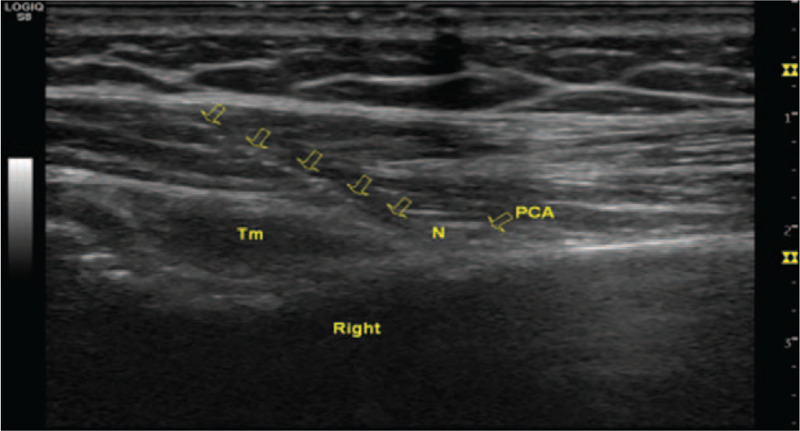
Axillary nerve block in quadrangular space under the guidance of ultrasound. N = axillary nerves, PCA = posterior humeral circumflex artery, Tm = Teres minor.
